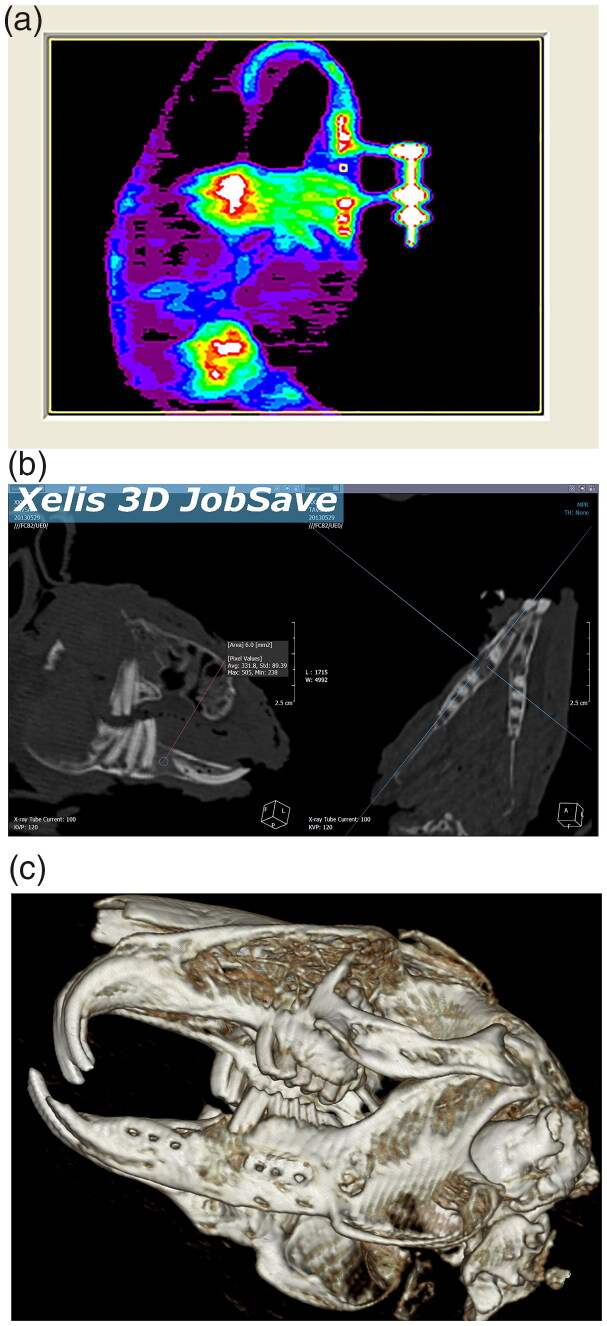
(A) DEXA. (B,C) Three-dimensional (CT) images showed healthly distracted bone and unilateral crossbite.
